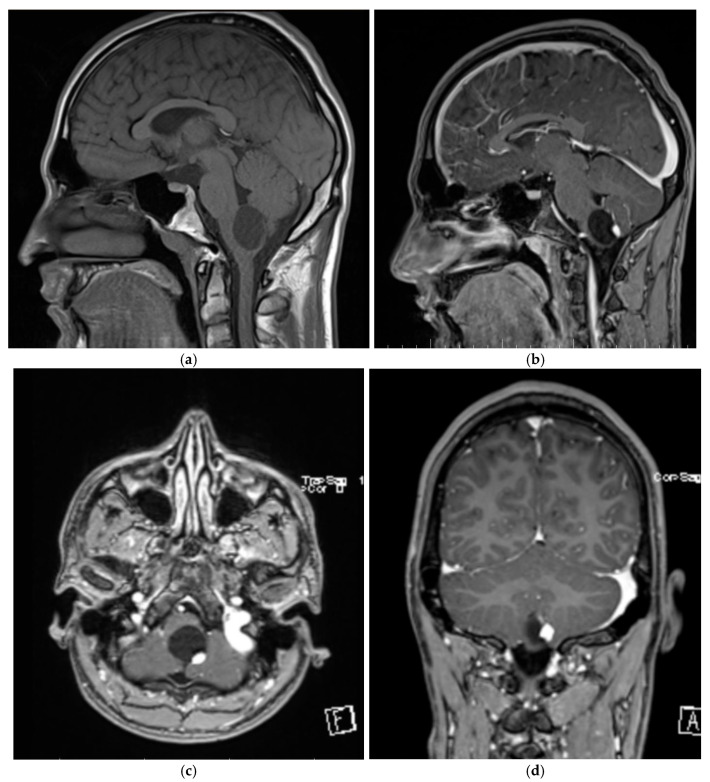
Preoperative brain MRI of patient three revealing a brain stem hemangioblastoma. (a) Sagittal section, native T1-weighted sequence, revealing a heterogeneous tumor process located at the craniospinal junction in the posterior part of the medulla oblongata, with cystic and solid, isopointense, well-defined composition. (b) Sagittal section, T1-T postcontrast sequence, revealing the intense and homogeneous gadolinium grip of the mural nodule. (c) Axial section, T1 sequence postcontrast. (d) Coronal section, T1 sequence postcontrast.
